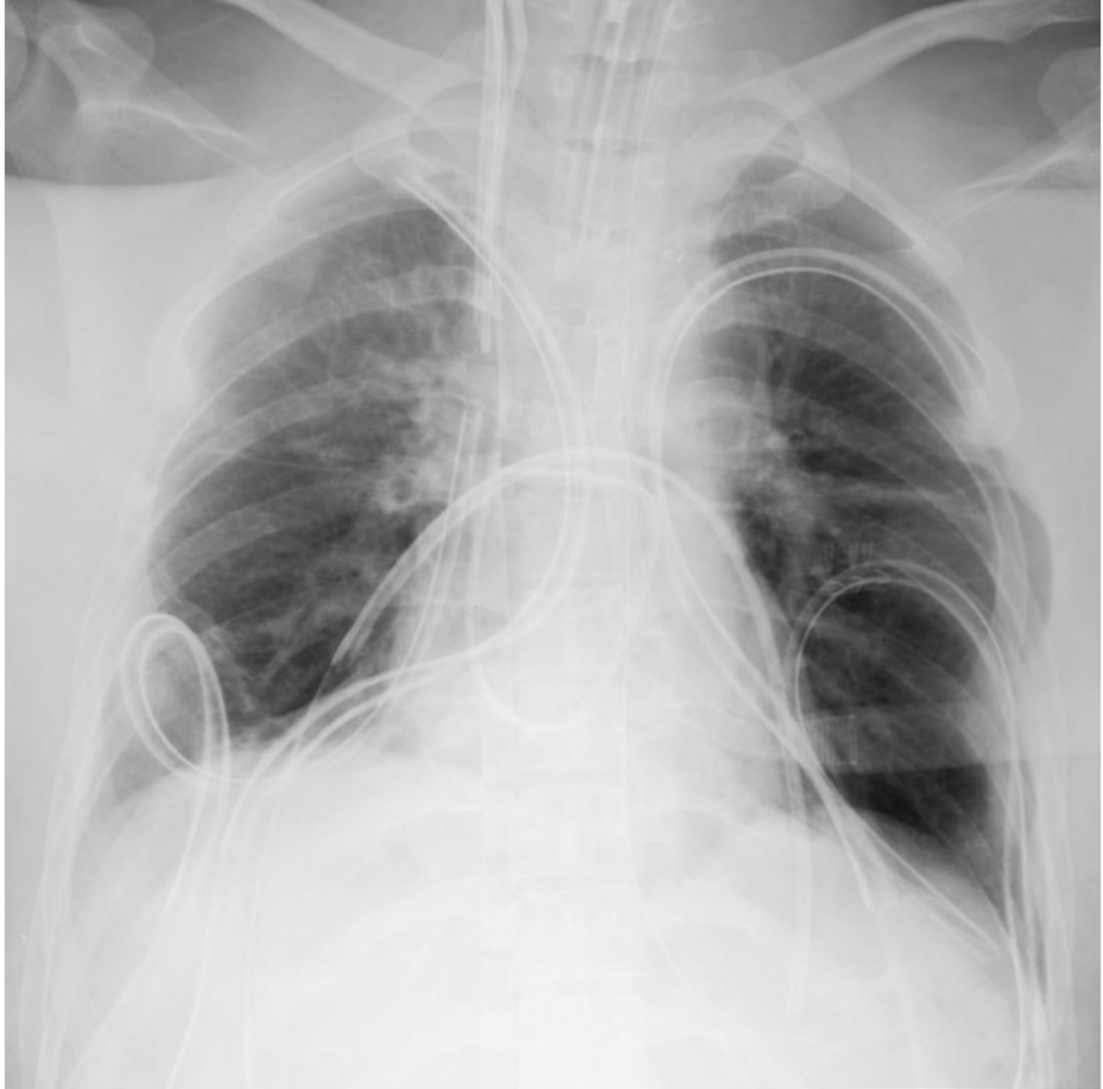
Postoperative chest radiography. A chest radiograph obtained after LTx showing ground-glass opacity in the right upper lung field
